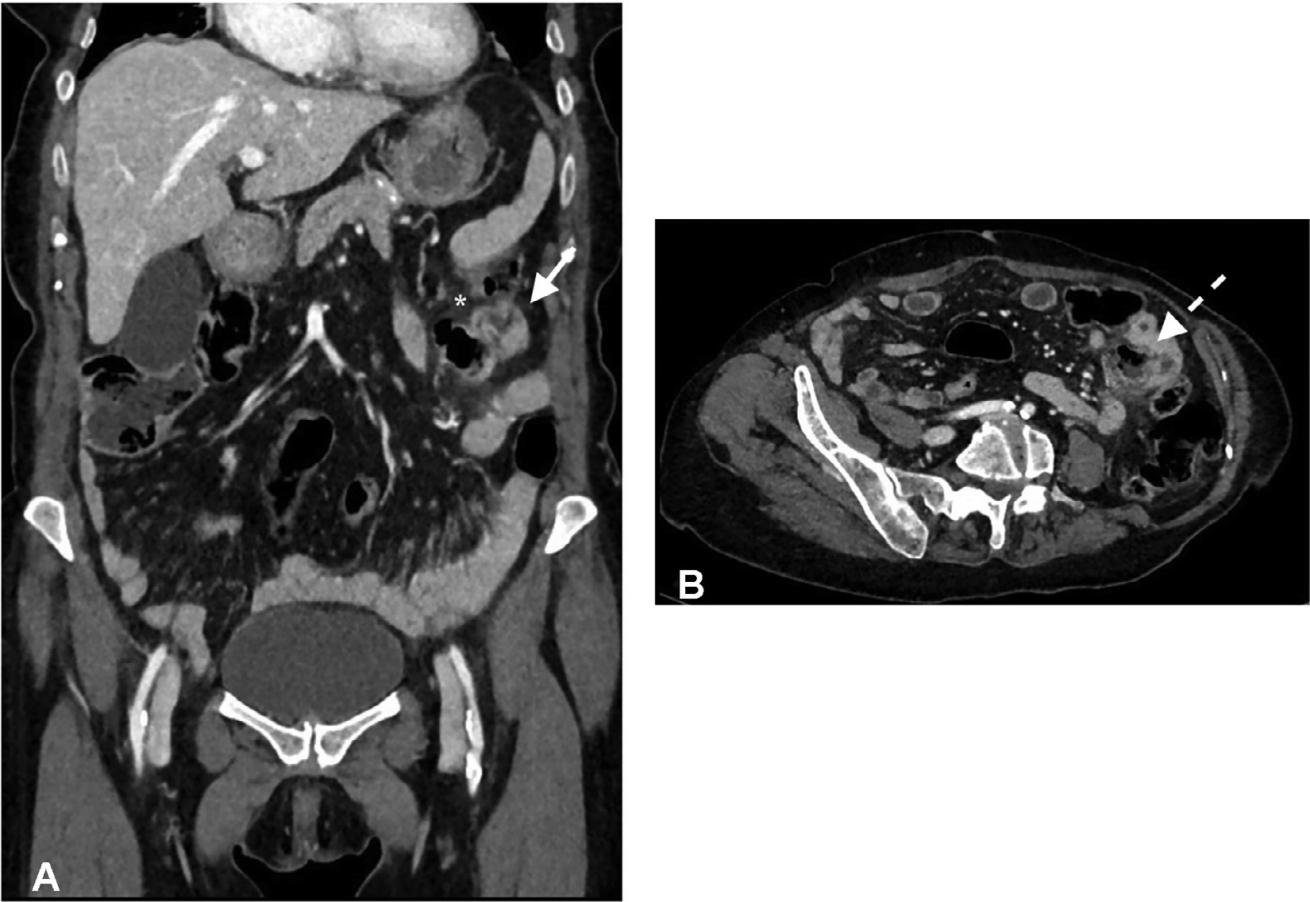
(A and B) Coronal and para-axial CT contrast images (parenchymal phase). The thickened jejunum loop is distinctly evident with the out-pouching diverticula sac on the mesenteric side (white arrow) with perilesional fat stranding (asterix) (A). On para-axial image, the diverticular neck is clearly visible (dashed arrow) (B).
